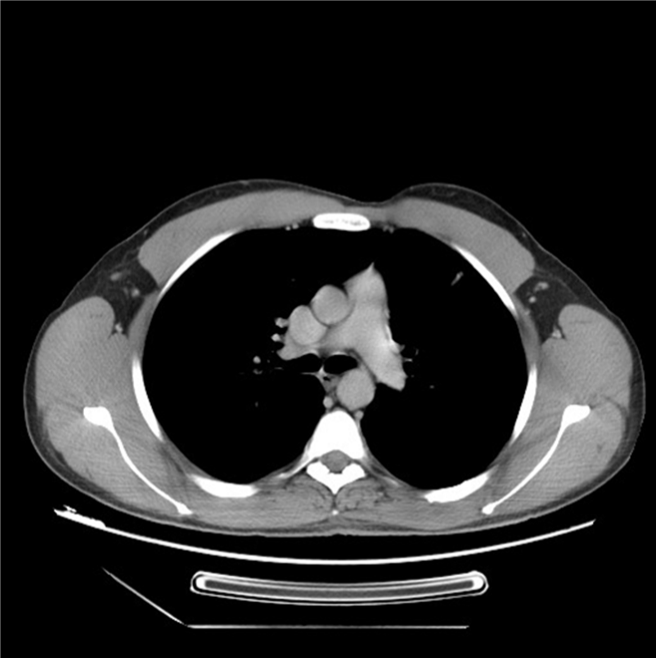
Computed tomography scan of the chest: complete response 5 years after diagnosis.
